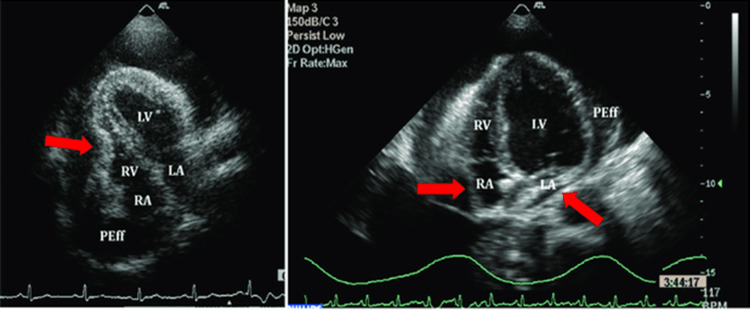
Right ventricular collapse on echocardiogram Source: Ref. [19].
